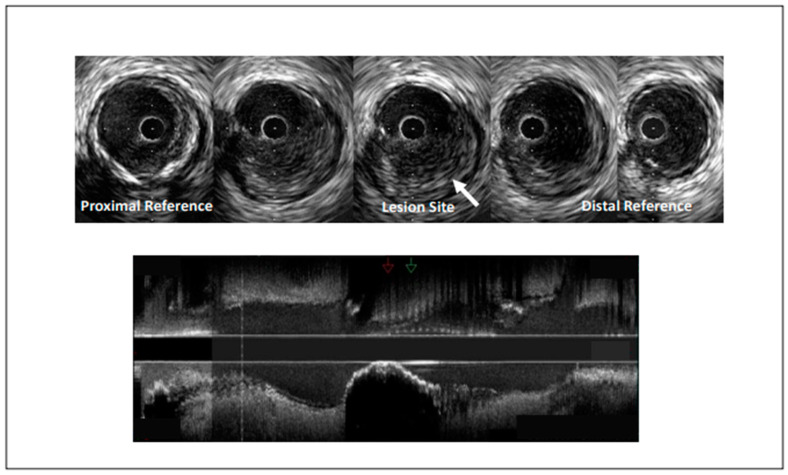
Intravascular ultrasound demonstrating coronary artery stenosis most likely due to plaque erosion.
